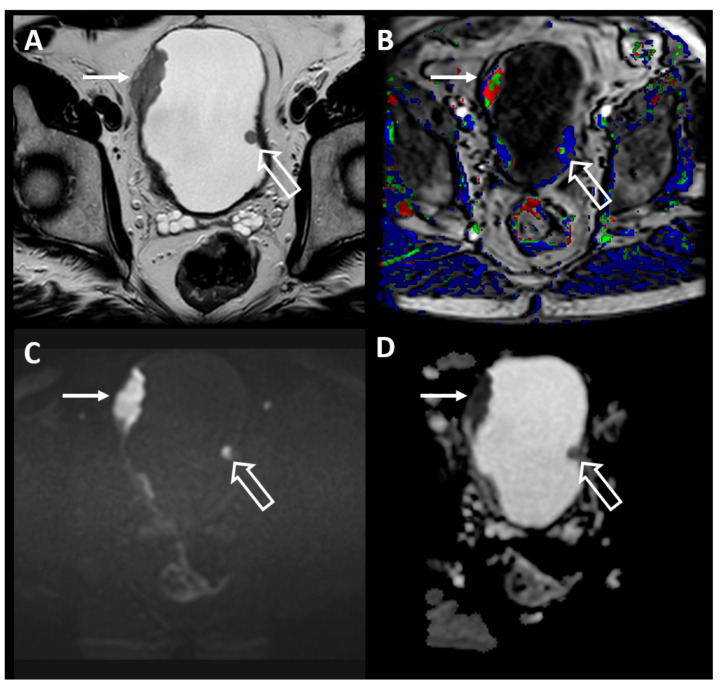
Axial T2-weighted (T2W) (A), dynamic-contrast enhanced (B), b2000 diffusion-weighted (C), and apparent diffusion coefficient (D) images of the pelvis in a patient with known multifocal bladder cancer. There are multiple intraluminal masses, one of which (closed arrow) clearly invades through the bladder wall and is consistent with muscle-invasive bladder cancer (MIBC), while the other polypoid mass (open arrow) does not disrupt the T2 hypointense bladder wall, consistent with non-muscle-invasive bladder cancer (NMIBC).
